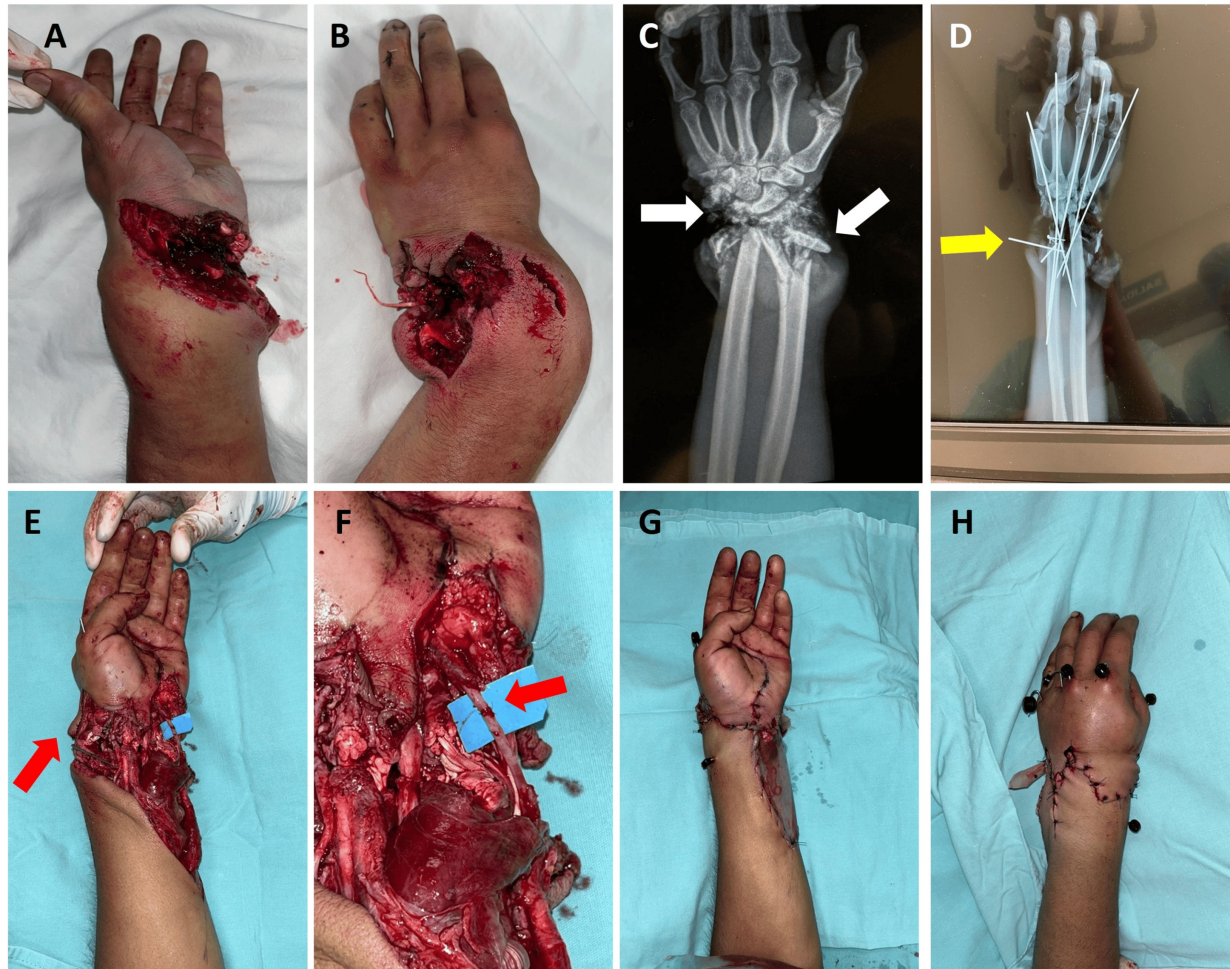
Pre- and post-surgery clinical and X-ray images and treatments in the initial surgery (case 3). (A and B) Preoperative volar and dorsal photograph. (C) Preoperative X-ray with carpal and distal forearm fractures (white arrows). (D) Fracture fixation (yellow arrow). (E and F) Radial and ulnar revascularization (red arrows). (G) Volar wound closure. (H) Dorsal wound closure.
